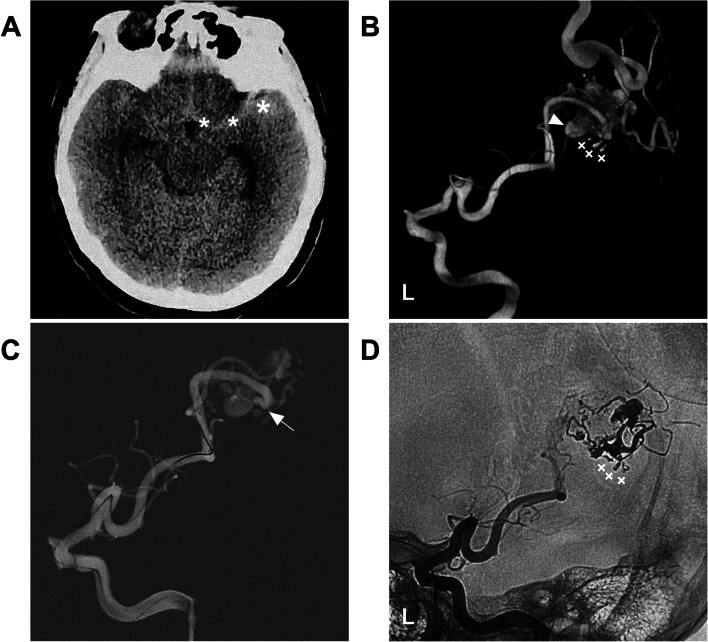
EVT targeting weak structures of BAVMs. A CT shows subarachnoid hemorrhage (asterisks) in the left Sylvian fissure. B Three-dimensional reconstruction angiogram of the left ICA shows that the BAVM is supplied by the M3 segment with aneurysmal structures (forks) and a venous dilation (arrowhead). C Roadmap navigation angiogram shows the microcatheter (arrow) accessed to the BAVM. D Unsubtracted angiogram of the left ICA showing Onyx casting in the aneurysmal structures (forks). Abbreviations: BAVM: brain arteriovenous malformation, CT: computed tomography, EVT: endovascular treatment, ICA: internal carotid artery, MCA: middle cerebral artery, M3: third segment of the MCA
